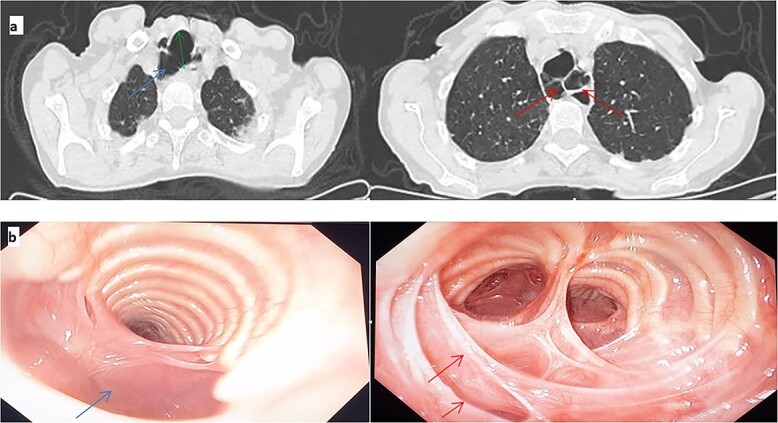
(a) Thoracic CT scan from the upper sections, a marked dilatation of the trachea with an estimated AP (anteroposterior) diameter of 32 mm (green double arrows) and tracheal diverticula (blue arrows) and septa (red arrows). (b) Bronchoscopy. Dilated trachea, diverticula (blue arrows) and septa (red arrows).
